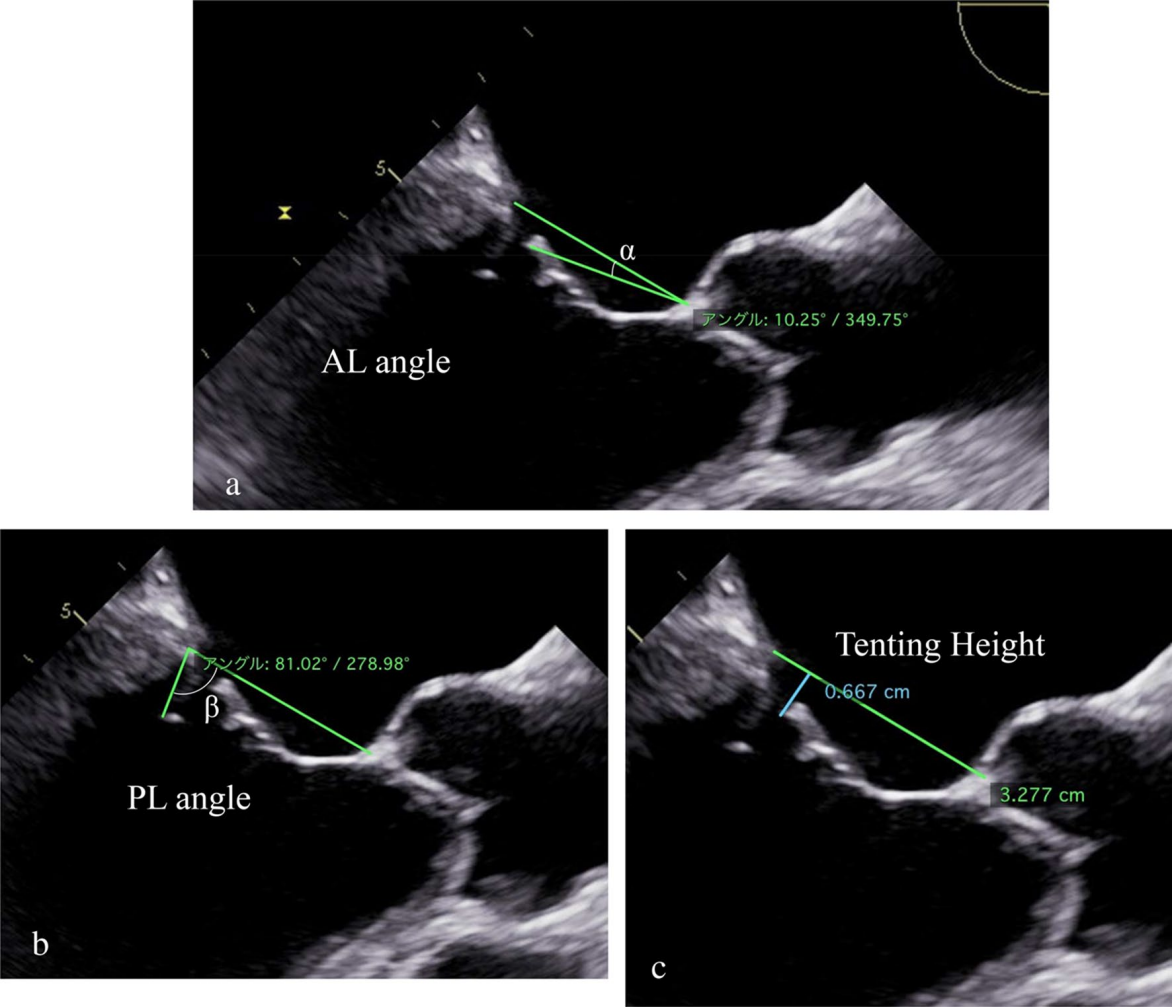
Preoperative transesophageal echocardiography at a long-axis view. a The α indicates the anterior mitral leaflet tethering angle (AL angle). b The β indicates the posterior mitral leaflet tethering angle (PL angle). c Tenting height was defined by the distance between the point of leaflet coaptation and the annular plane (blue line).
